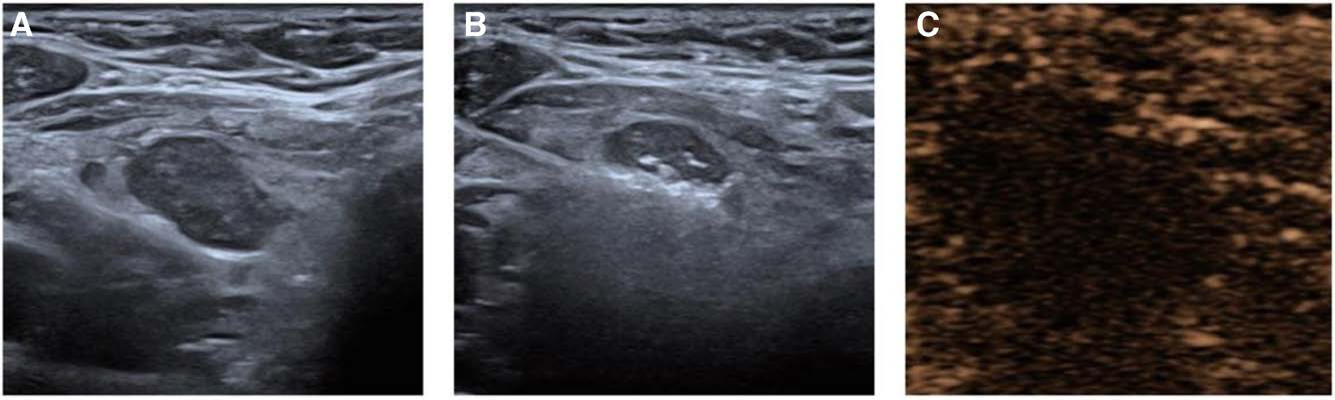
Ultrasound images of patients during ablation. (A) Normal saline was injected between the parathyroid lesion and important surrounding structures to form a fluid isolation area. (B) Ultrasound-guided microwave ablation of parathyroid nodule. (C) Contrast-enhanced ultrasound was performed immediately after the operation, showing no enhancement within the lesion, which proved that the ablation was complete.
